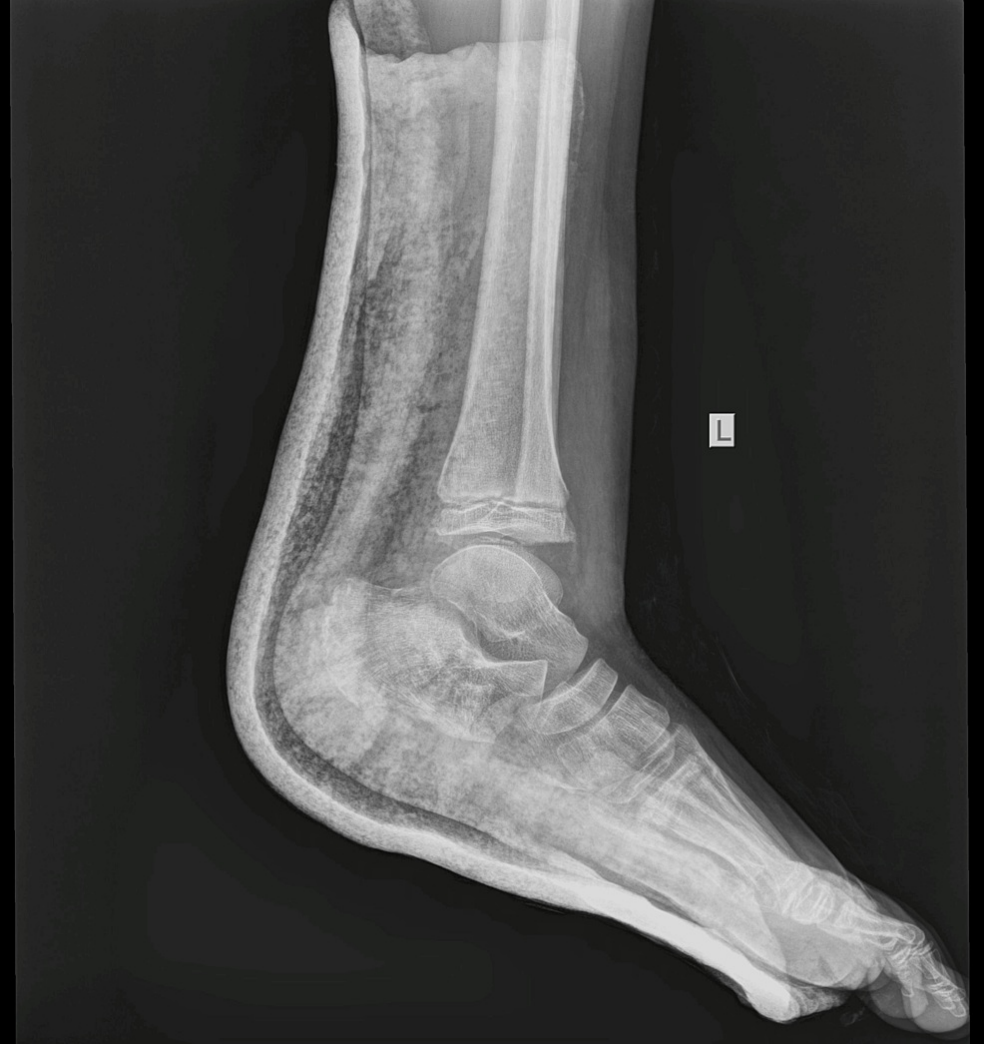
X-ray lateral, after cast immobilization
